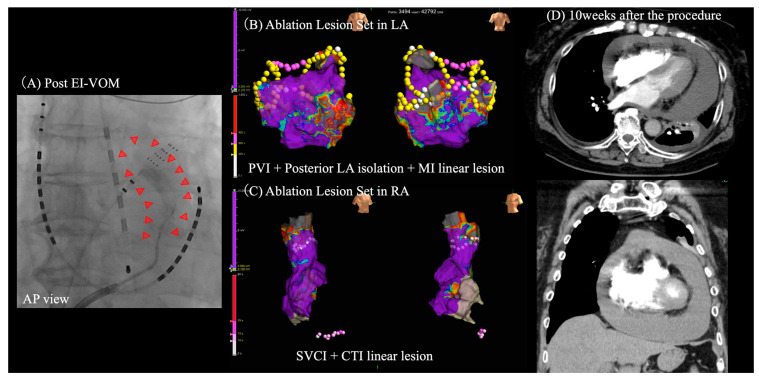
An example case of delayed cardiac tamponade. A 78-year-old female underwent EI-VOM (A), followed by PVI, MI line, and posterior LA isolation in the LA (B). Additionally, she underwent a CTI linear lesion and SVCI in the RA (C) to treat long-standing persistent AF. Initially, the outcomes were positive, with a significant removal of AF symptoms. During follow-up visits at 3 and 8 weeks post-procedure, the patient reported no specific symptoms, and her BNP level remained stable at <50 pg/mL. However, by the 9th week, she began experiencing shortness of breath, prompting her visit to the emergency department in the 10th week. On examination, she presented with mild hypotension (blood pressure 88/60) and sinus tachycardia (115 bpm). Her hemoglobin level remained consistent at 11 g/dL, but her BNP level had increased to 120 pg/mL. A CT scan revealed a large pericardial effusion (D). Subsequent pericardiocentesis drained 1100 mL of pale red fluid from the pericardial sac. AF, atrial fibrillation; AP, anteroposterior view; BNP, brain natriuretic peptide; CT, computed tomography; CTI, cavotricuspid isthmus; EI-VOM; ethanol infusion to the vein of Marshall; LA, left atrium; MI, mitral isthmus; PVI, pulmonary vein isolation; RA, right atrium; RAO, right anterior oblique view; SVCI, superior vena cava isolation.
